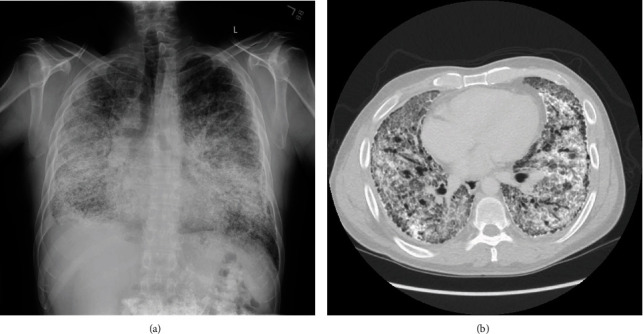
Radiologic findings consistent with PAM. (a) Chest X-ray demonstrating diffuse bilateral interstitial hyperdensities with bibasilar predominance consistent with severe interstitial lung disease. (b) Transverse view of chest CT showing diffuse ground-glass opacities, peripheral cystic change, and diffuse areas of fine calcification.
